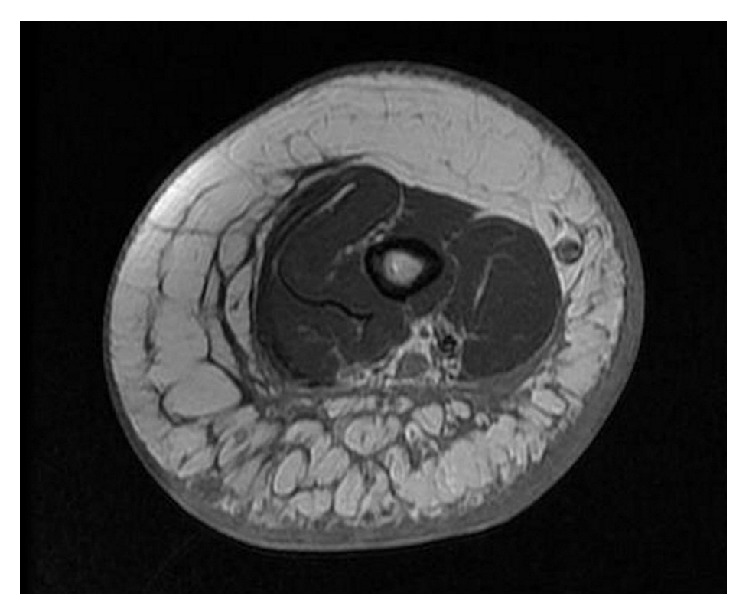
MRI of the soft parts of the upper left limb objectivating edematous infiltration of the fatty tissue with the presence of nodular formation opposite the brachial artery.
